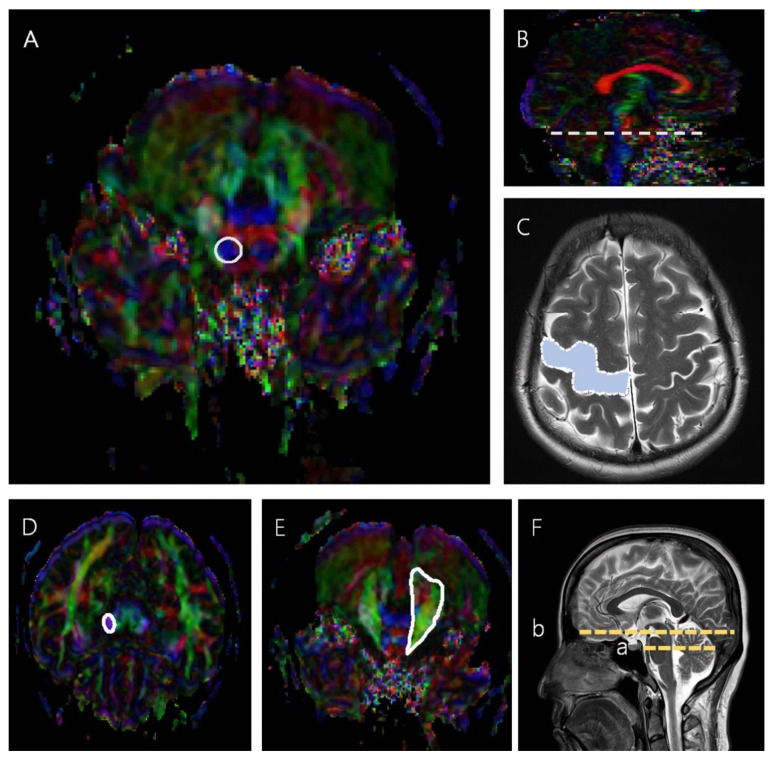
The seed and target ROIs used to reconstruct the CST and CPCT. (A) The region inside the solid line represents the seed ROI in the CST, mid-pons, in an axial color map. (B) The dotted line represents the mid-pons level in a sagittal color map. (C) The blue region in the dotted line represents the target ROI in the CST in an axial brain MRI scan. (D) The region in the solid line represents the target ROI in the CPCT at the cerebral peduncle in an axial color map. (E) The region in the solid line represents the seed ROI in the CPCT at the middle cerebellar peduncle, mid pons, in an axial color map. (F) (a) is the slice level of the seed ROI, and (b) is the slice level of the target ROI in a sagittal brain MRI scan.
